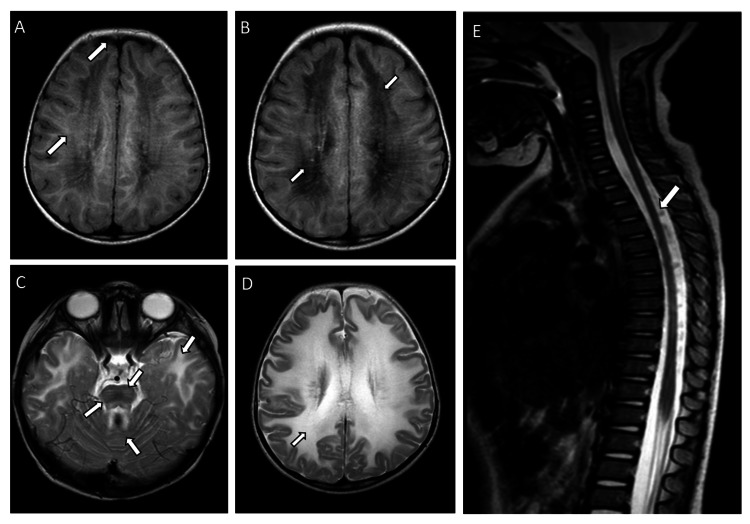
Axial flair brain and spine magnetic resonance imaging (MRI) of patient 1 at the level of the centrum semiovale (A) Brain MRI at the age of 21 months showing diffuse bilateral symmetrical periventricular white matter changes extending to the subcortical U fibers (arrows). (B, C) Repeated brain MRI at the age of two years showing further progression of the white matter disease with involvement of the brain stem. (D) T2-weighted brain MRI image showing the progression of the disease (arrow). (E) T2-weighted spinal cord MRI showing diffuse atrophic appearance (arrows).
